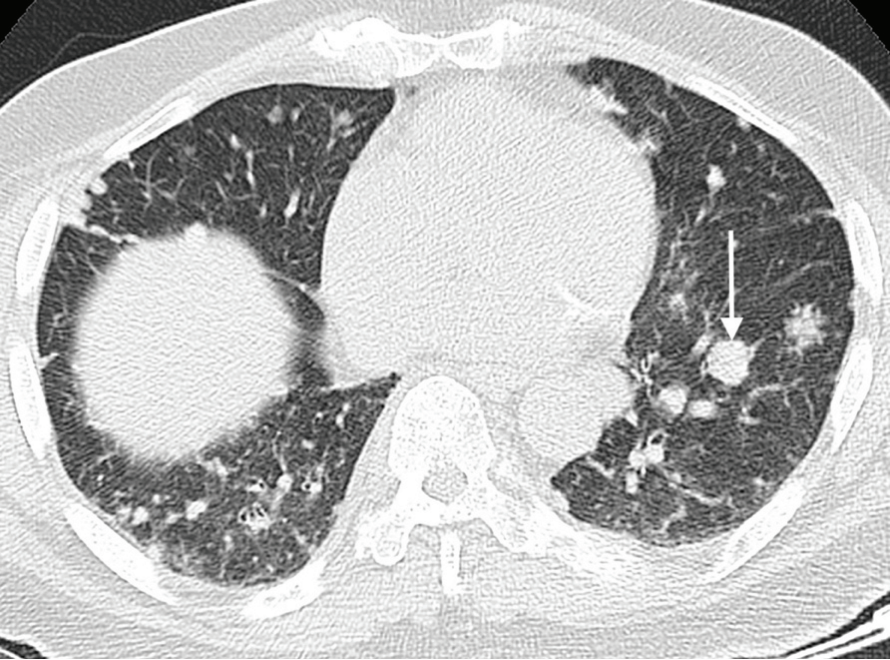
Axial CT chest (pulmonary window) of an 80-year-old man with known advanced prostate cancer demonstrating several pulmonary metastases with a predominantly nodular pattern; largest nodule indicated on the left side (arrow).
